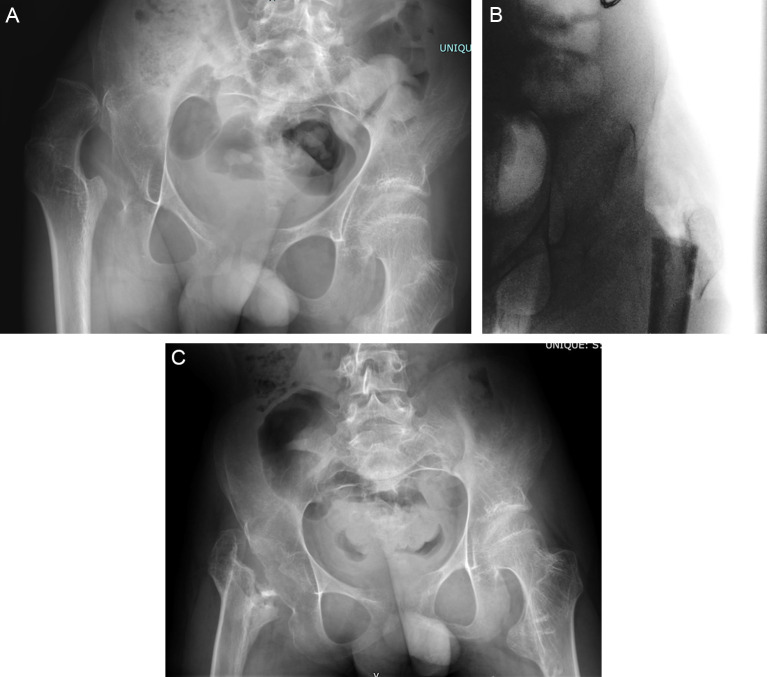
(A) Preoperative radiograph of 18-year-old male, GMFCS III/IV with right hip pain and difficulty with weight bearing and activities of daily living. Note the asphericity of the femoral head and skeletal maturity indicating that remodeling of the femoral head here was not going to be substantive. (B) Intraoperative radiograph demonstrating the relationship between the trochanteric fragment and the femoral shaft. These pieces of bone are transfixed with #2 Fibrewire sutures. (C) Final 1-year radiograph of hip salvage intervention. This young man has returned to weight bearing without pain and his overall function has improved significantly. Note the appropriate station of the proximal femur without significant migration and pelvic support configuration.
